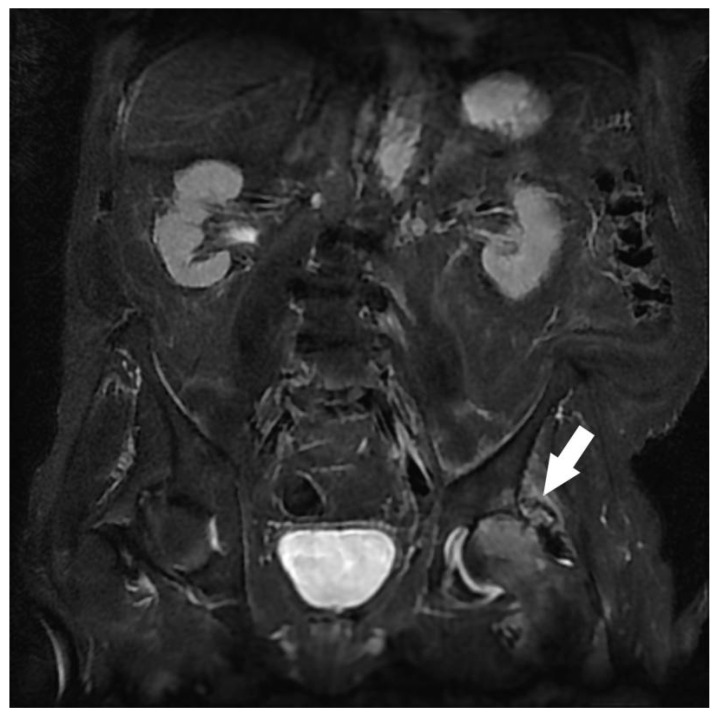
Assessment of left sciatica in an 87-year-old man. Coronal STIR MRI. Left coxo-femoral hypersignal predominant on the femoral head, narrowing of the joint space mainly supero-external, significant joint effusion, and degenerative hypertrophy of the labrum. Congestive left hip osteoarthritis (white arrow).
